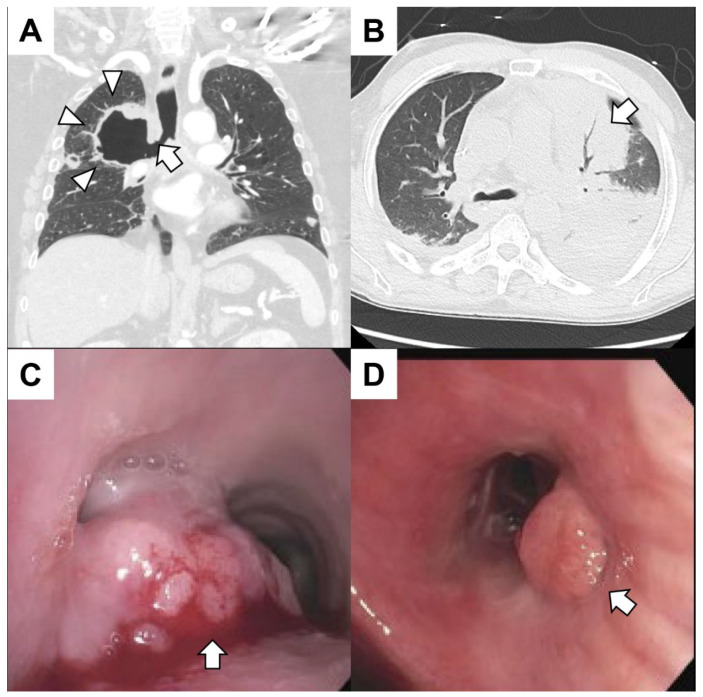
(A) A patient with metastatic lung cancer treated with radiation 1 month prior to the right upper lobe presented with hemoptysis. The coronal CT of the chest revealed a cavitary lesion (arrowheads) contiguous with the right mainstem bronchus (arrow). The patient expired from massive hemoptysis shortly after presenting to the emergency center. (B) A patient with leukemia with pancytopenia (white blood cells 0.0 × 10^9^/L platelets 14,000 × 10^9^/L) and fever presented with hemoptysis. The patient had sepsis with bacteremia. A chest CT revealed consolidation in the left upper lobe (arrow) with air bronchograms. (C) A patient with gastroesophageal junction cancer presented with dyspnea and mild hemoptysis. Bronchoscopy revealed a mass at the main carina obstructing 90% of the left mainstem and 20% of the right mainstem. The mass (arrow) was large, exophytic and friable. The tumor was debulked using rigid bronchoscopy, and argon plasma coagulation was used to achieve hemostasis. Biopsies were consistent with metastatic disease. (D) A patient with metastatic colorectal cancer had dyspnea and mild hemoptysis. The patient had mucopurulent and blood-tinged secretions emanating from the right middle lobe. An endoluminal tumor mass (arrow) obstructing the superior segment of the left lower lobe was found. The hemoptysis resolved after treatment of a respiratory infection.
